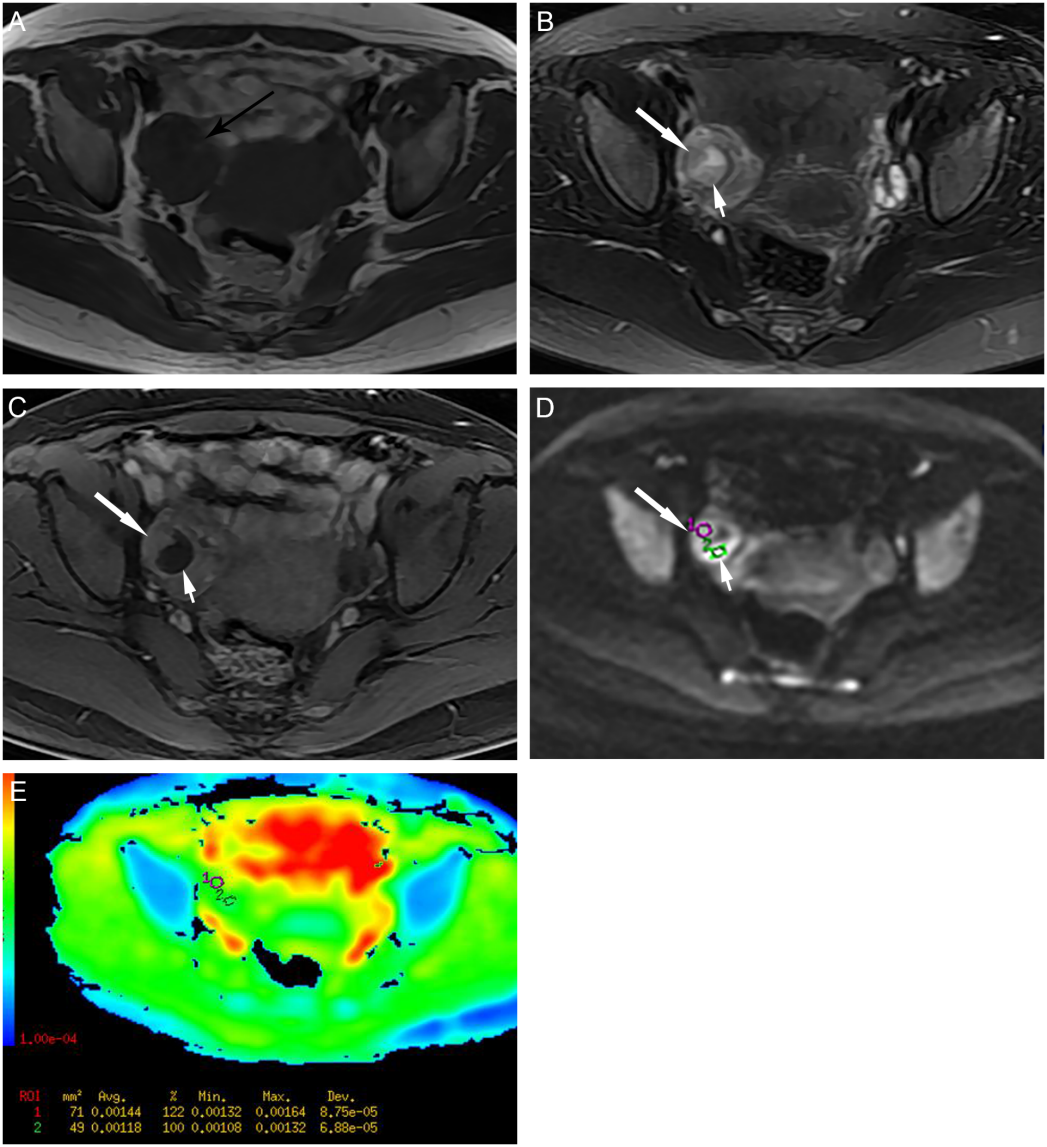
A 37-year-old woman with right ovarian abscess, showing a complex mixed cystic and solid appearance. (A) Axial T1-weighted image shows a intermediate signal intensity mass in the right side of the pelvic cavity. (B) Axial T2-weighted image indicates that the mass has intermediate signal intensity and a central cystic component (high signal intensity area). (C) Axial contrast-enhanced MR image shows a mixed cystic and solid mass with thick wall, presenting marked rim-enhancement. The soft-tissue signal intensity area (pseudosolid) on the T2-weighted image shows no enhancement (short white arrow). (D-E) Axial DW image shows the pseudosolid area is high signal intensity, compatible with purulent cavities with low ADC value. The enhancing solid component (long white arrow) also shows high signal intensity on DW image, mimicking an adnexal maligancy (pseudotumor area), with a relatively high ADC value (circle 1 ADC = 1.440 × 10^−3^ mm^2^/s; circle 2 ADC = 1.180 × 10^−3^ mm^2^/s).
